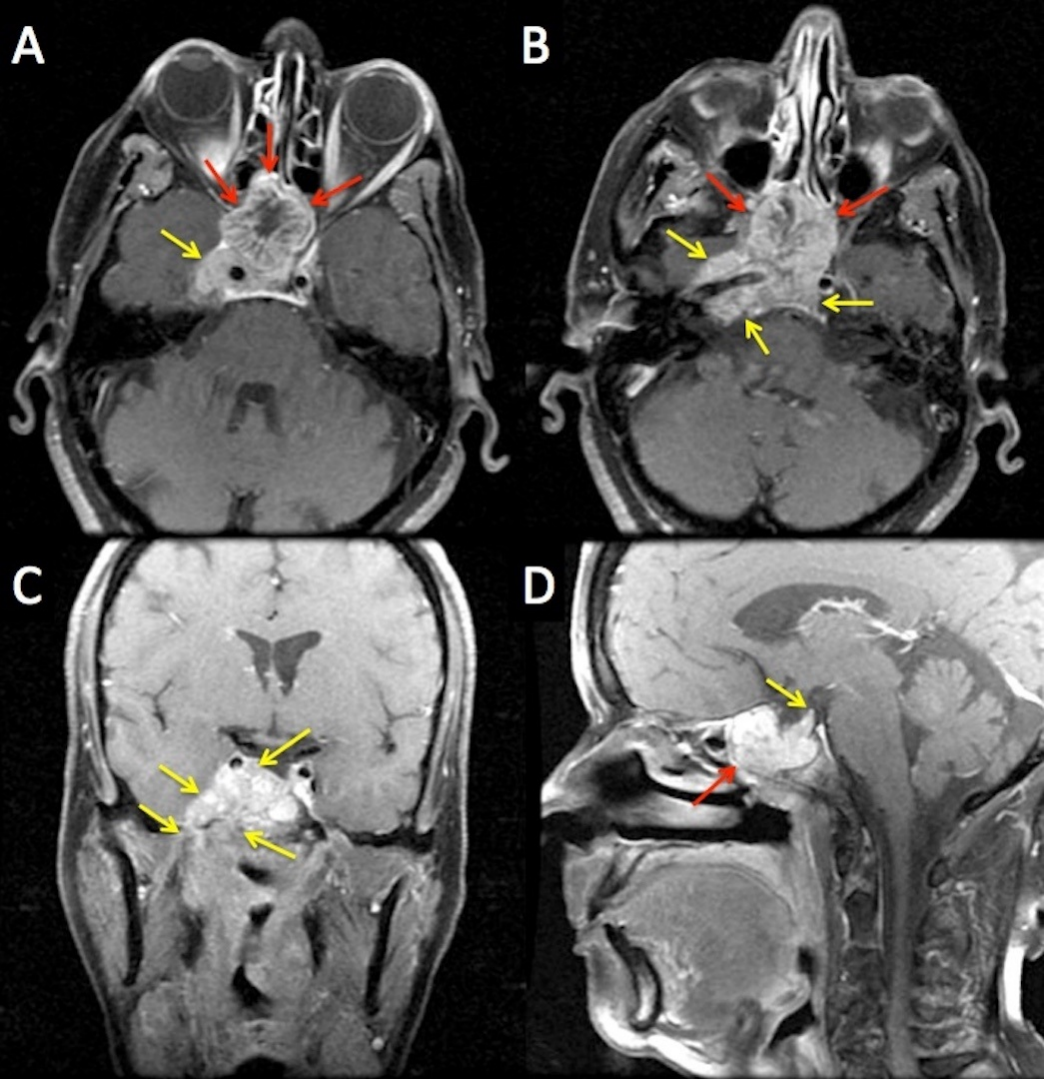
Representative MRI images at diagnosis, showing the large nasopharyngeal mass with intracranial extension Large, exophytic, primary tumor (red and yellow arrows) in nasopharynx (red arrows) invading into the right foramen ovale and the cavernous sinus (yellow arrows). The tumor is in close proximity to the normal brain, optic nerve, optic chiasm, and globes. A and B - axial images showing the nasopharyngeal mass (red arrows) and the intracranial extent into the cavernous sinus and the proximity of the tumor to the brainstem (yellow arrows), C - coronal image showing the superior and inferior extent of the intracranial portion of the tumor (yellow arrows), D - sagittal image showing tumor involvement of the clivus (red arrow) and the intracranial extent of the tumor, in proximity to the brain stem (yellow arrow).
